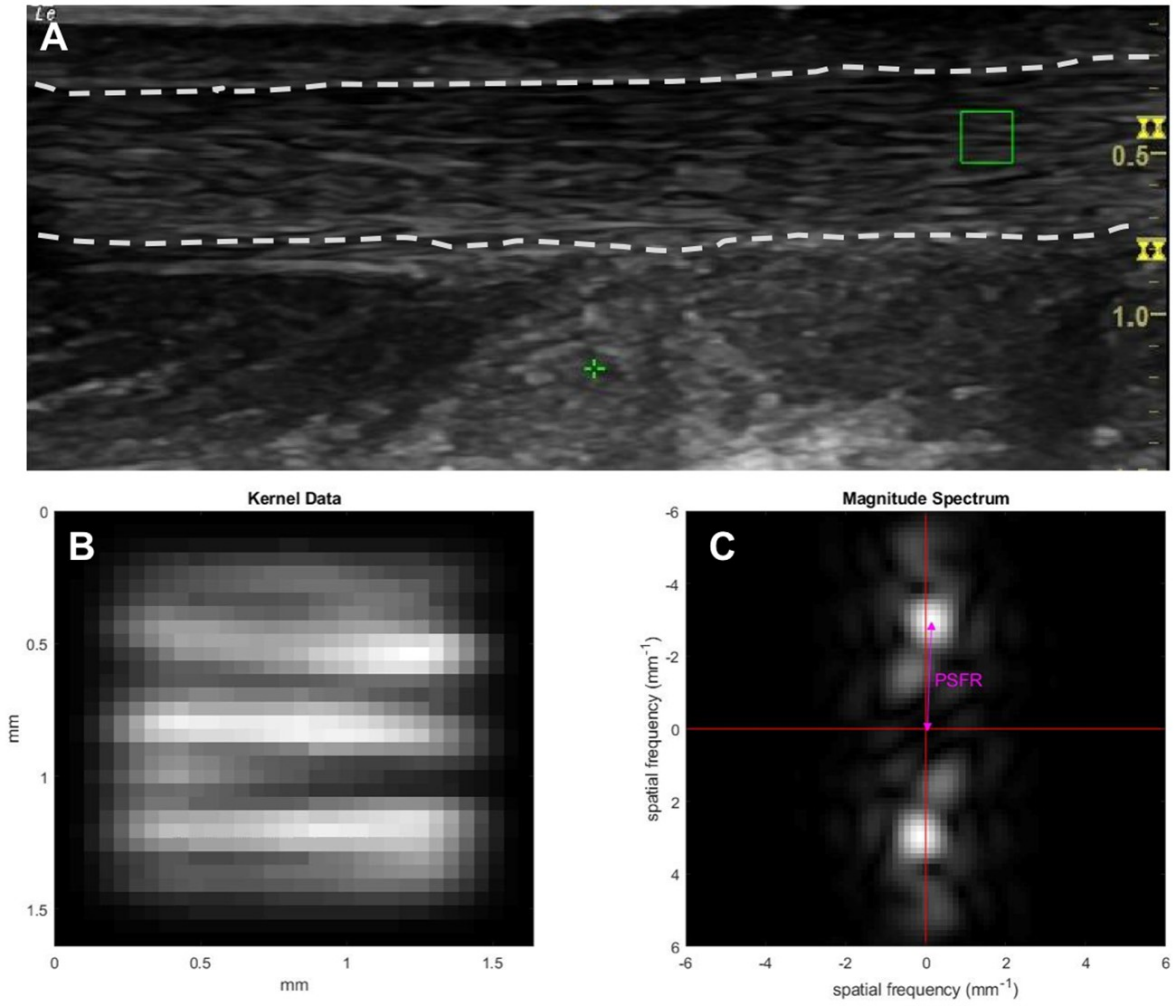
Frequency analyses of an Achilles tendon ultrasound image. (A) Original B-mode image with a sample kernel (green rectangle); (B) the zoomed data and spectrum of the kernel; (C) corresponding frequency spectrum of the selected kernel with a double arrow denoting peak spatial frequency radius. Area between the two dashed lines on the B-mode image is the Achilles tendon.
